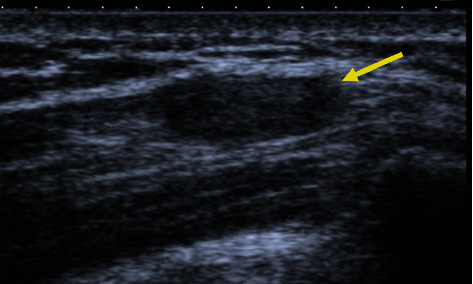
Ultrasonography of the right shoulder identified a hypoechoic nodular formation (yellow arrow) in the posterior deltoid, with approximately 8 × 10 × 13 mm in size.
